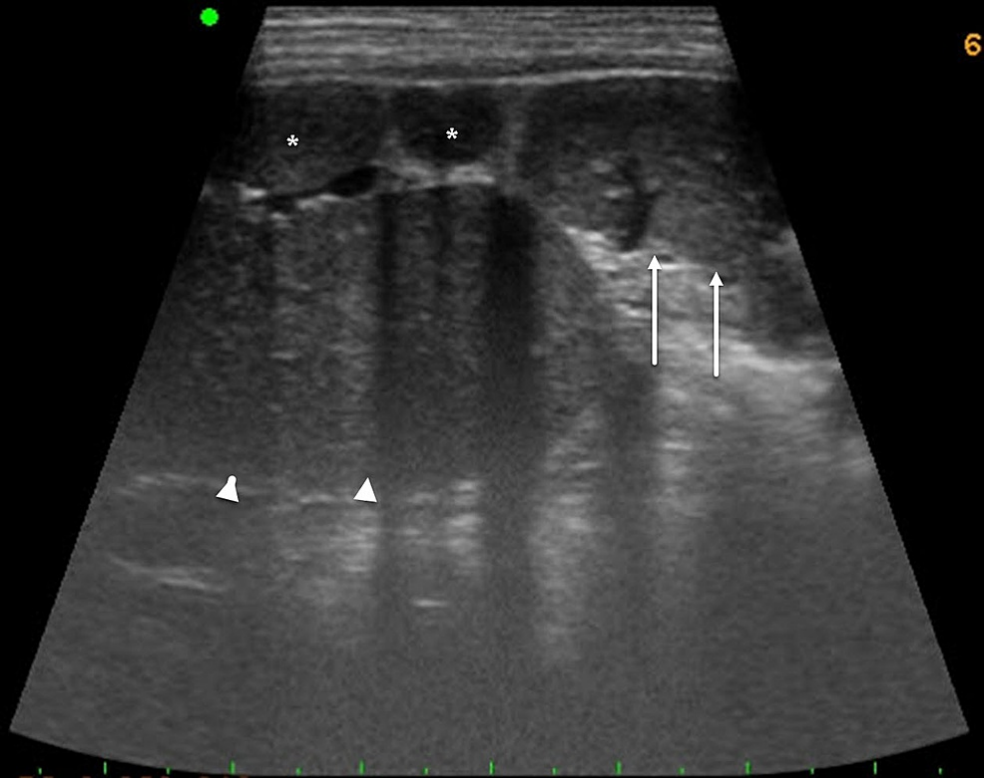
Oblique sections on gray-scale sonography of a five-year-old child suffering from heterotaxy syndrome acquired over the left upper abdomen, slightly posteriorly, revealing multiple spleens (asterisk), extension of enlarged right lobe of liver (arrowheads), and a malpositioned inferiorly displaced left kidney (arrows).
